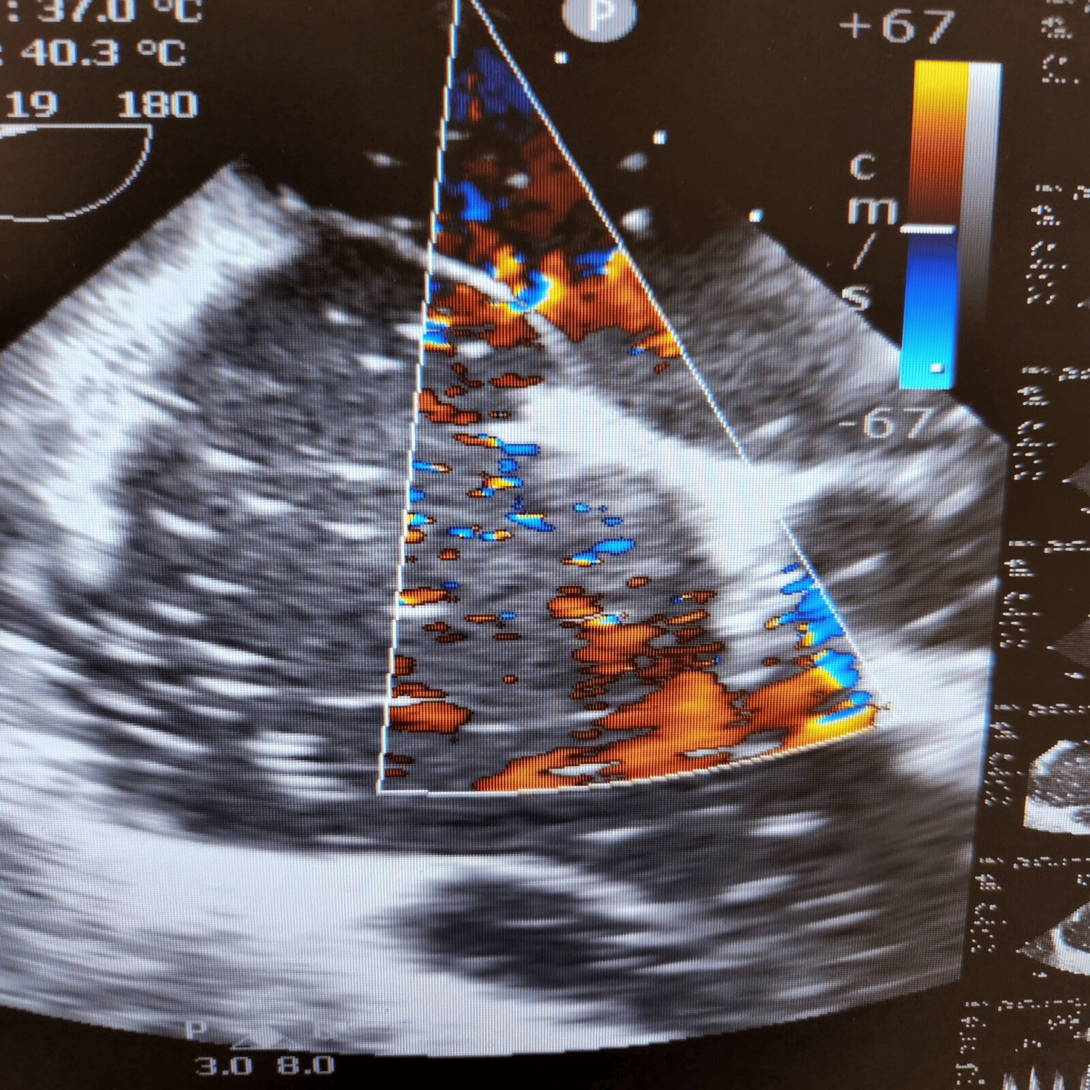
Gas bubbles seen in the left ventricle (seen as white spots) in a 2D echo (echocardiogram)
